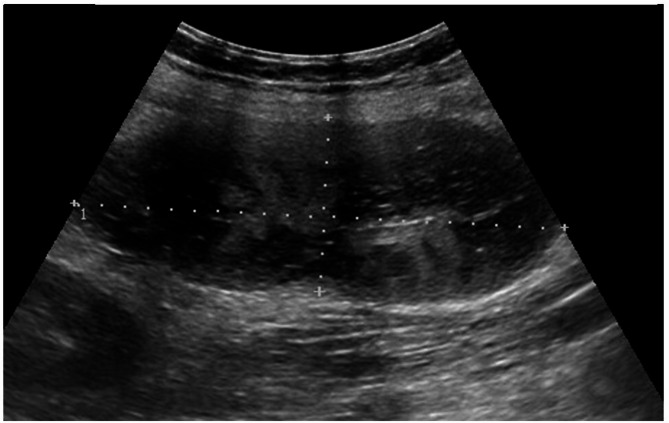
US scan shows a hypoechoic mass measuring 11 × 4 cm, with sharp margins, identified in the left flank - left iliac fossa, with no blood flow at Power-Doppler integration
